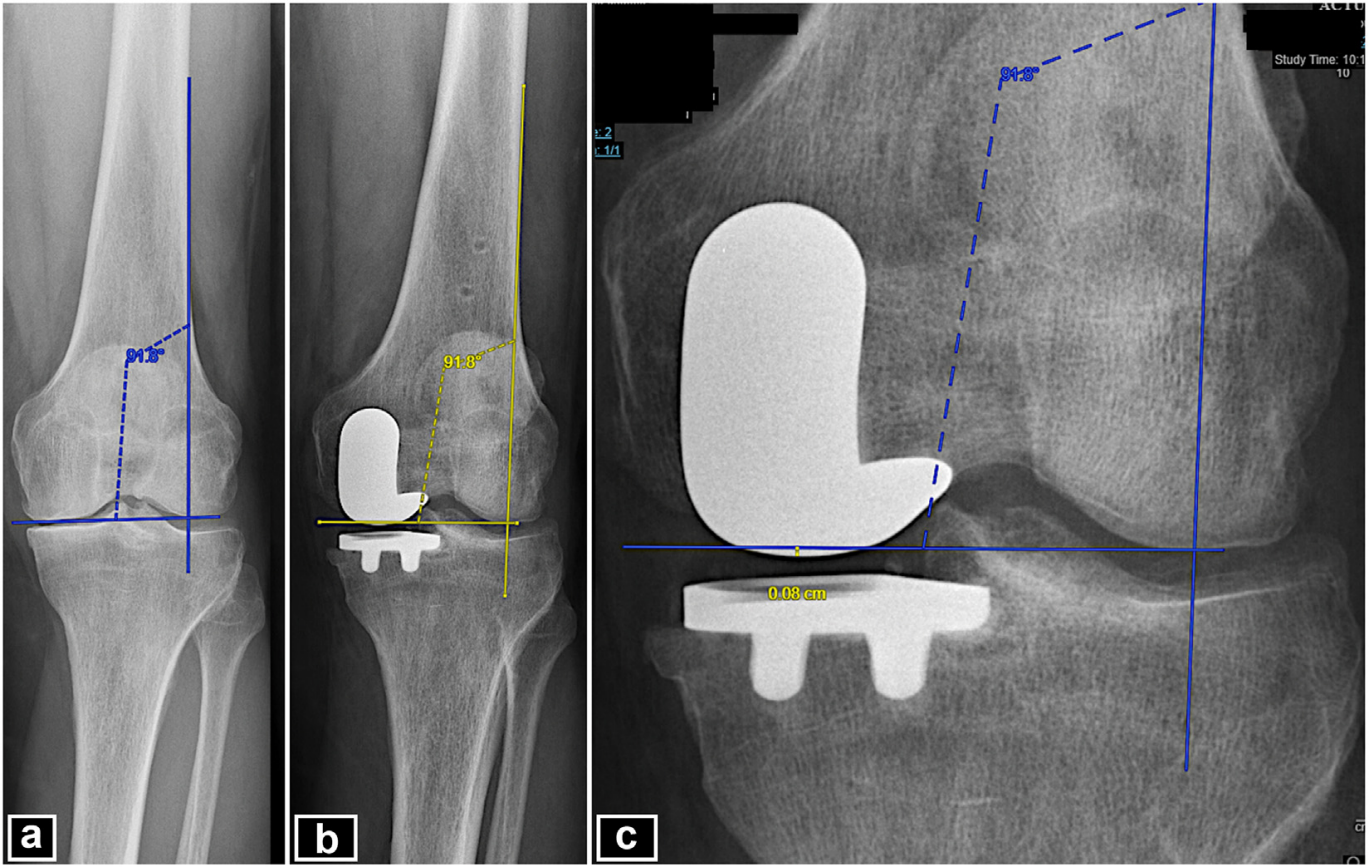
Preoperative and postoperative AP radiographs of left knee. (a) Measurement of joint line height using Herry’s method [17]. (b and c) Measurement of change in the height of the joint line using Herry’s method [17]. A joint line distalization of 0.8 mm can be observed. The joint line is measured by the angle formed between a line tangential to the lateral femoral cortical bone and another line that runs through the most distal point of the femoral condyles in a preoperative AP knee radiograph. On postoperative x-rays, the same angle measured preoperatively is applied to the lateral femoral cortex and to the distal end of the lateral femoral condyle. The distance between the most distal point of the femoral component and this line is measured in milliliters and is defined as the change in height of the joint line, as described and validated by Herry et al [18].
